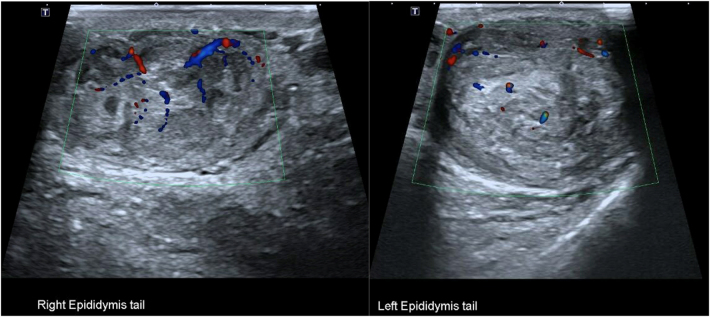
Ultrasound image of the epididymal tail lesions. The lesions could be located as arising from the epididymal tails and appearances were identical bilaterally. The lesions were well-defined and of mixed echogenicity with mild internal vascularity. No calcification or convincing whirl pattern was seen.
